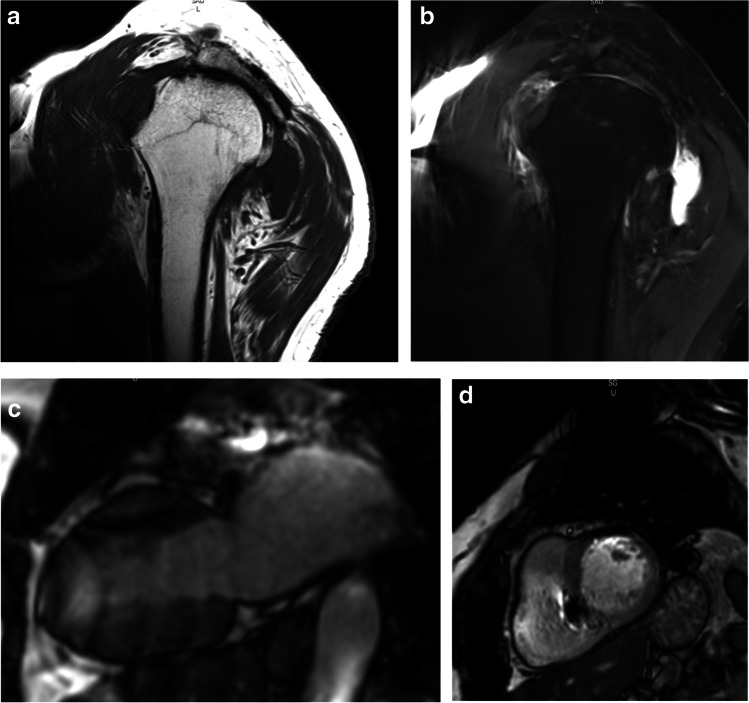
T1- (a) and T2-weighted (b) medium quality images of the left shoulder of an obese male patient performed on a 3 T Siemens equipment. Note presence of magnetic susceptibility artifacts on the left-hand side of both images. Balanced FFE (b-FFE) vertical long axis view of the heart (c) drawn from an examination performed in an overweight woman on a 3 T Philips equipment. Less artifacts are visible on the b-FFE short axis view (d) drawn from the same examination that was rated medium quality
